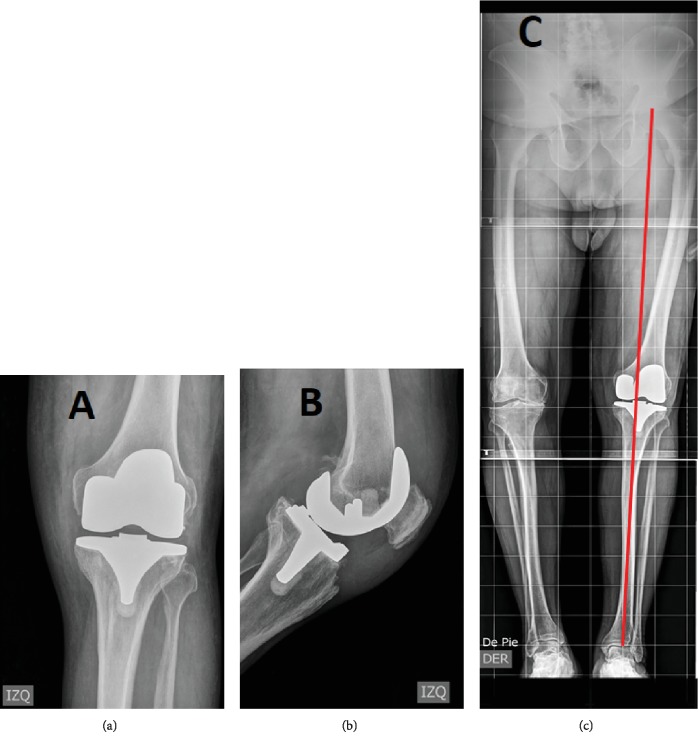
Left knee radiograph two years after embolization: (a) anterior-posterior left knee view, (b) lateral left knee view, and (c) lower anteroposterior limb view with a red line showing the mechanical axis.
